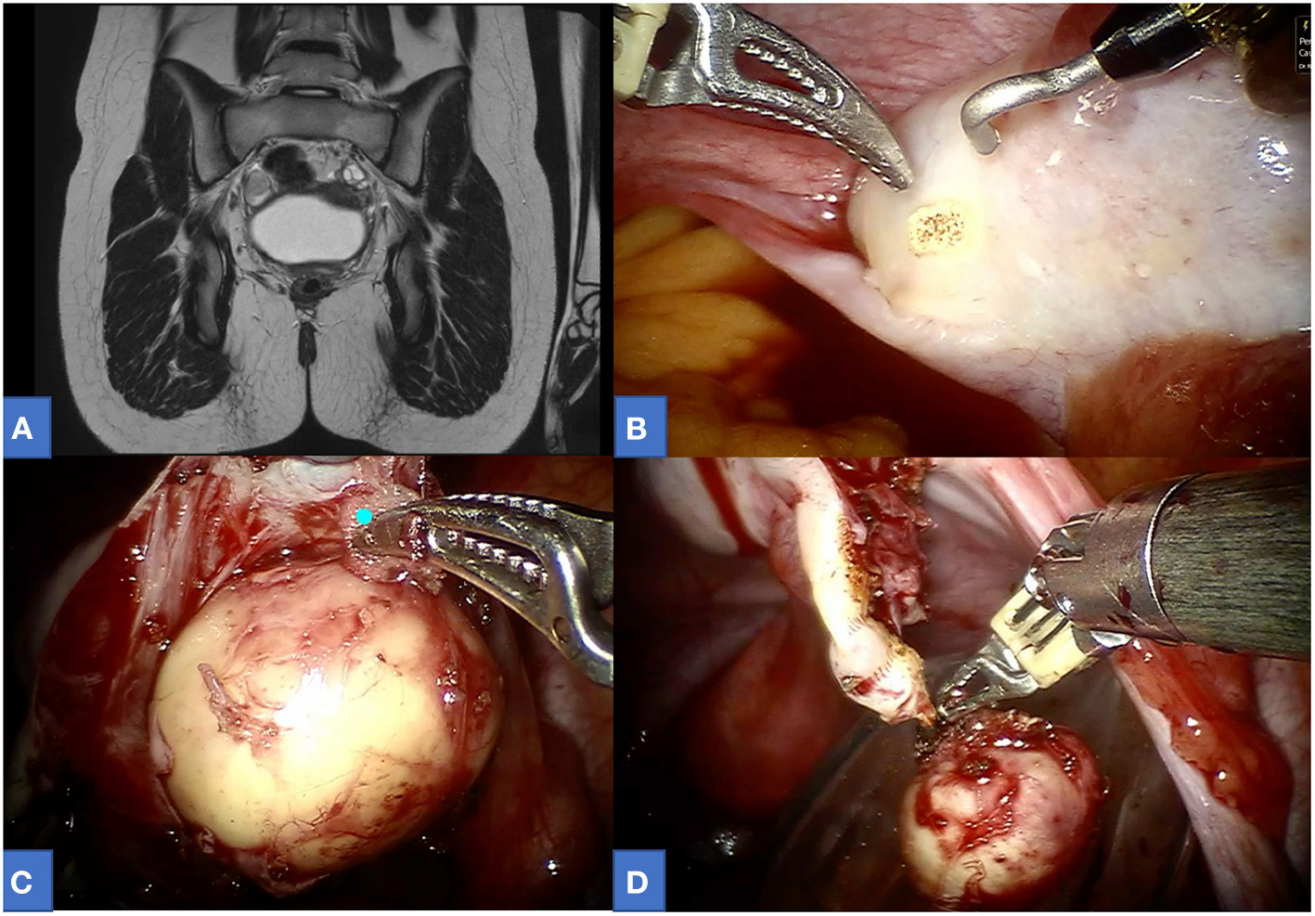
A 13-year-old affected by right ovarian mature teratoma. (A) Preoperative MRI. (B–D) Intraoperative view.
